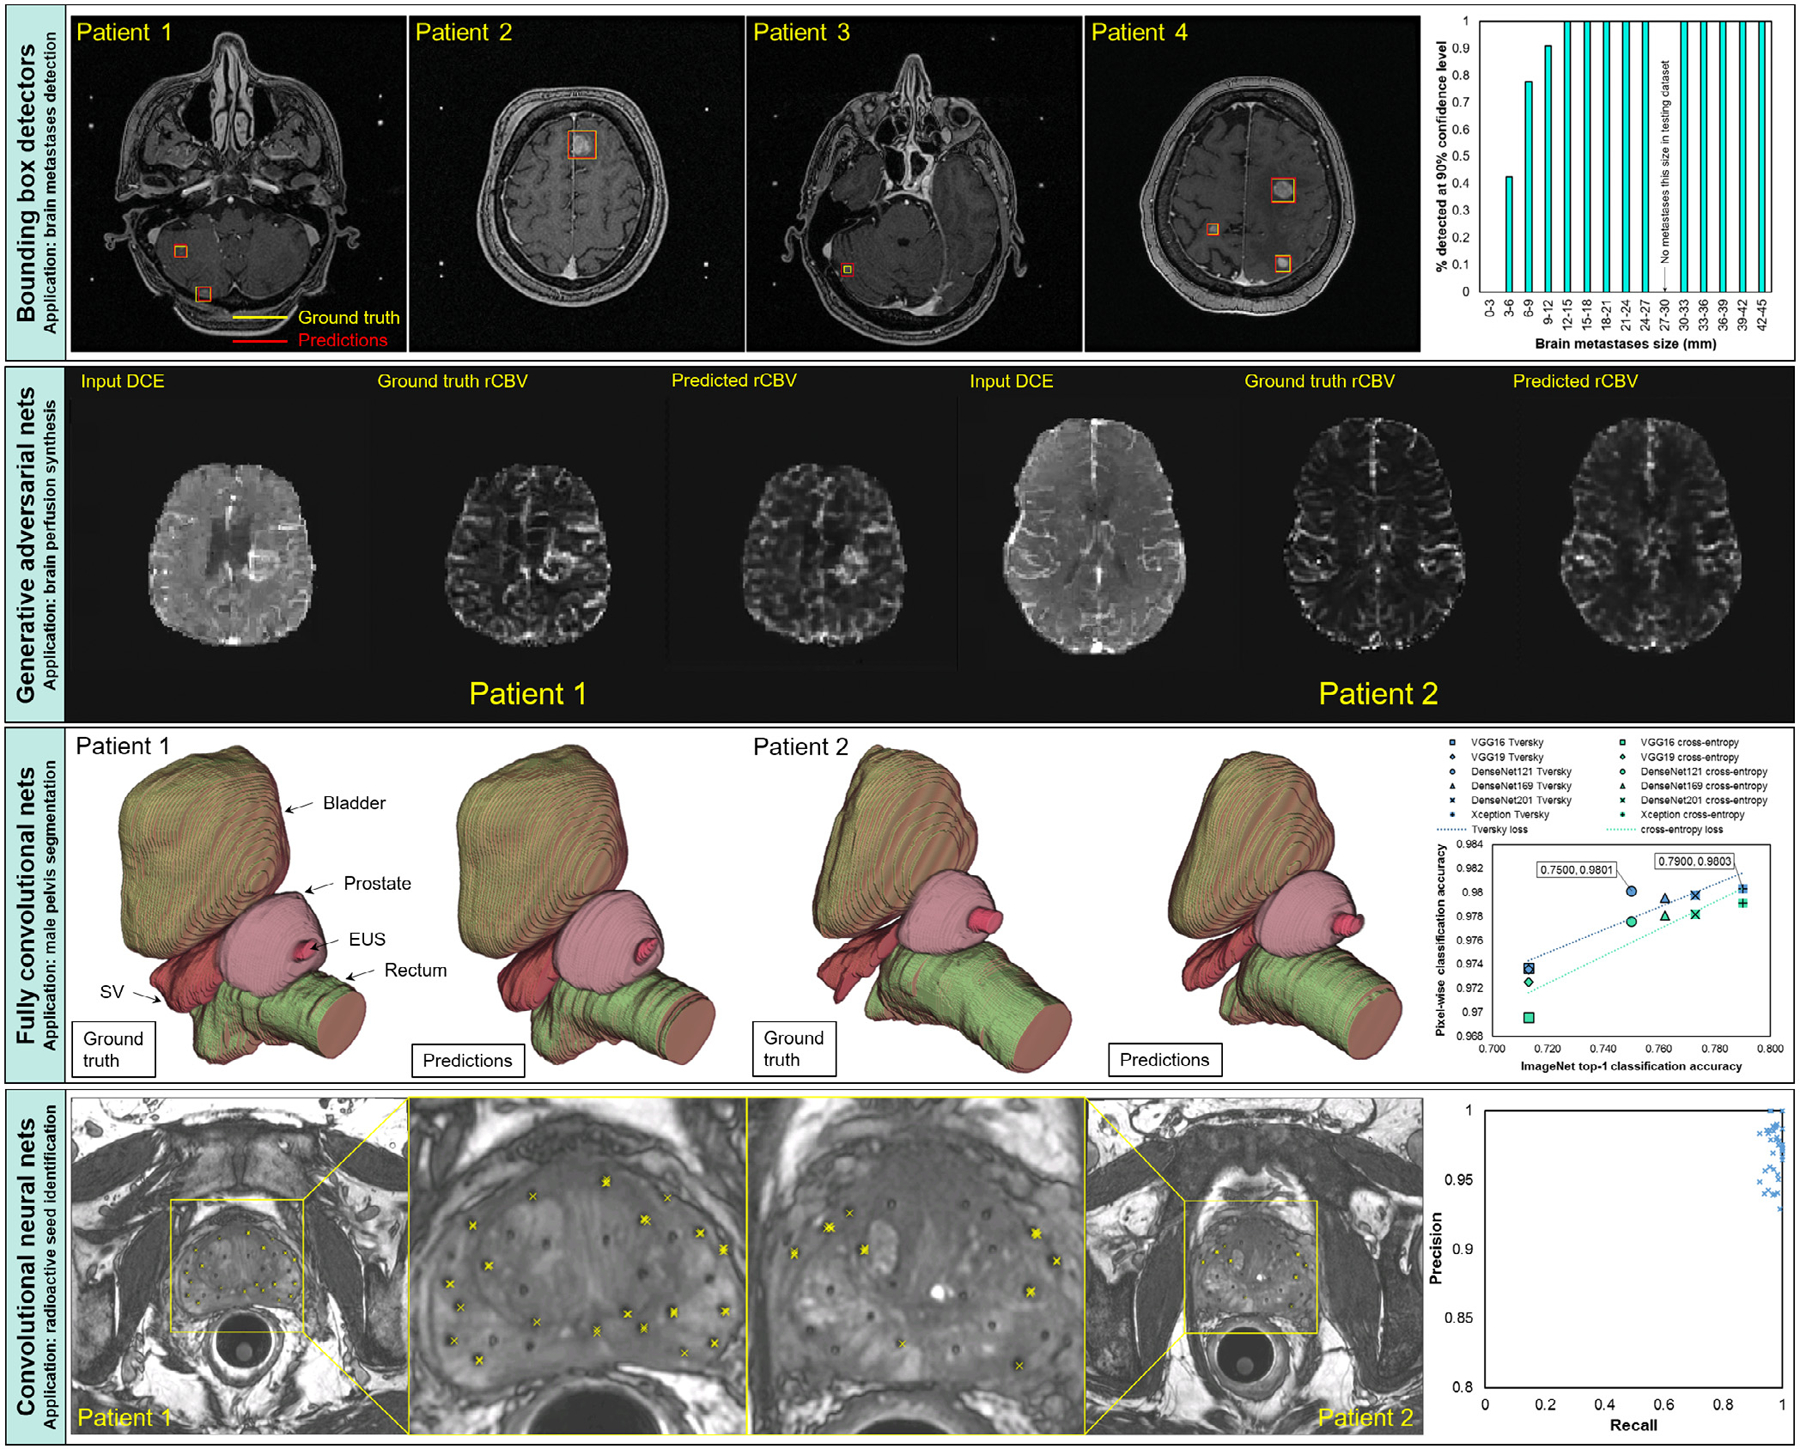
(First band, BBD) (Left) Example detection inferences for four patients with brain metastases. (Right) Detection sensitivity with respect to brain metastasis size at a 90% confidence threshold. (Second band, GAN) A comparison of true and synthetic rCBV maps generated from DCE-MRI. (Third band, FCN) (Left) Inferred radioactive seed locations for two representative patients. (Right) Precision versus recall for 20 test patients. (Fourth band, CNN) (Left) An example set of manual and inferred contours for a prostate cancer patient after brachytherapy seed implantation. (Right).
